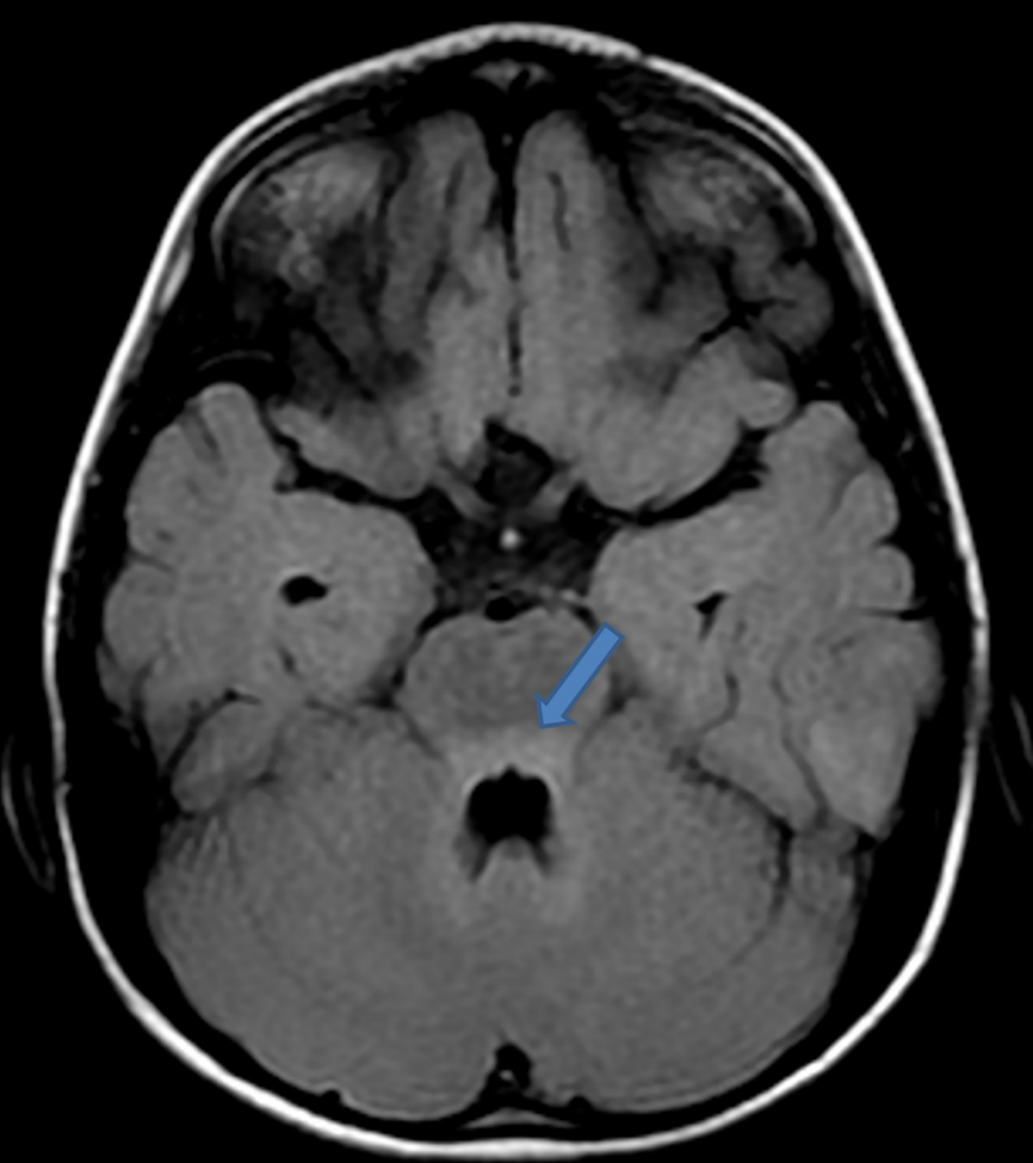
T2/FLAIR hyperintensities noted in posterior 1/3 of pons, pontomedullary junction, medulla oblongata, and posterior part of left temporal gyri.
